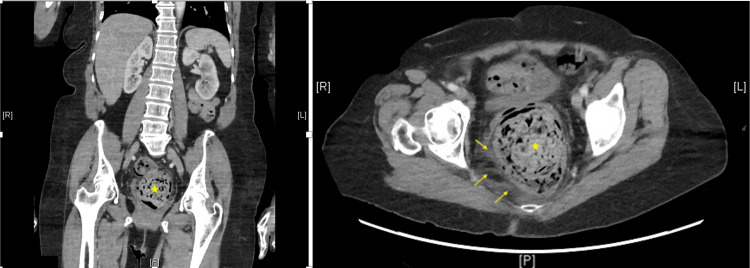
Coronal and axial views of CT scan with contrast of the abdomen and pelvis reveals large rectal stool burden (star) with mural thickening and perirectal inflammatory changes (arrows) CT: computed tomography
